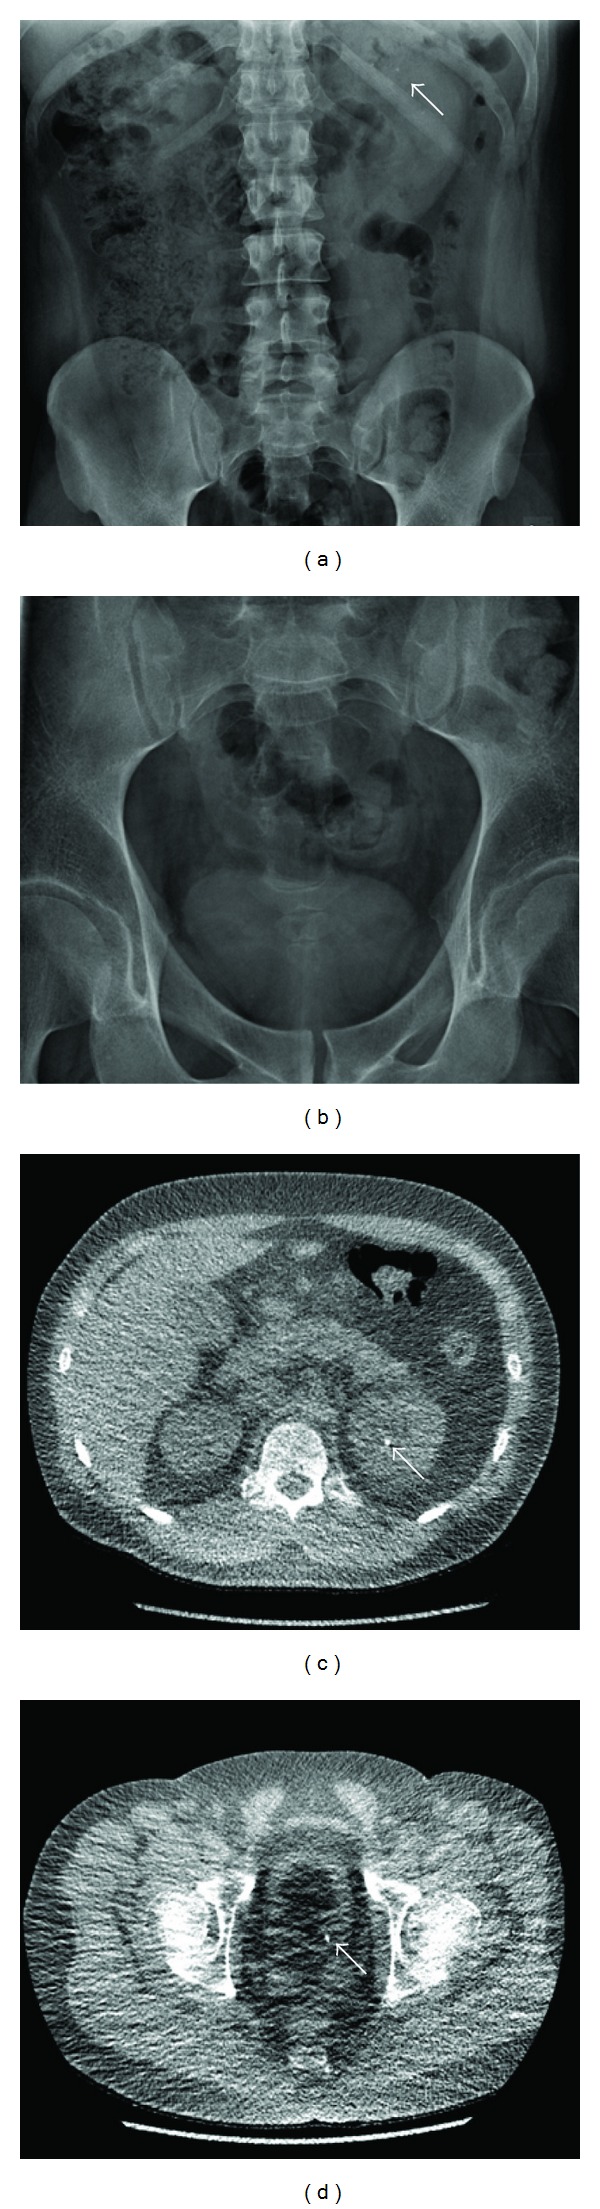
A 25-year-old male presenting with left flank pain. (a) Plain radiograph of the abdomen showing a possible renal calculus in the upper pole of the left kidney (estimated institutional dose ~0.7 mSv). The coned pelvic radiograph (b) does not demonstrate a calculus in the pelvis. Low-dose CT KUB (effective dose of 0.5 mSv) (c) and (d) clearly identifies a 5 mm calculus in the upper pole of the left kidney, as well as a 4 mm calculus, at the left ureterovesical junction. The latter was not seen on the plain radiograph.
